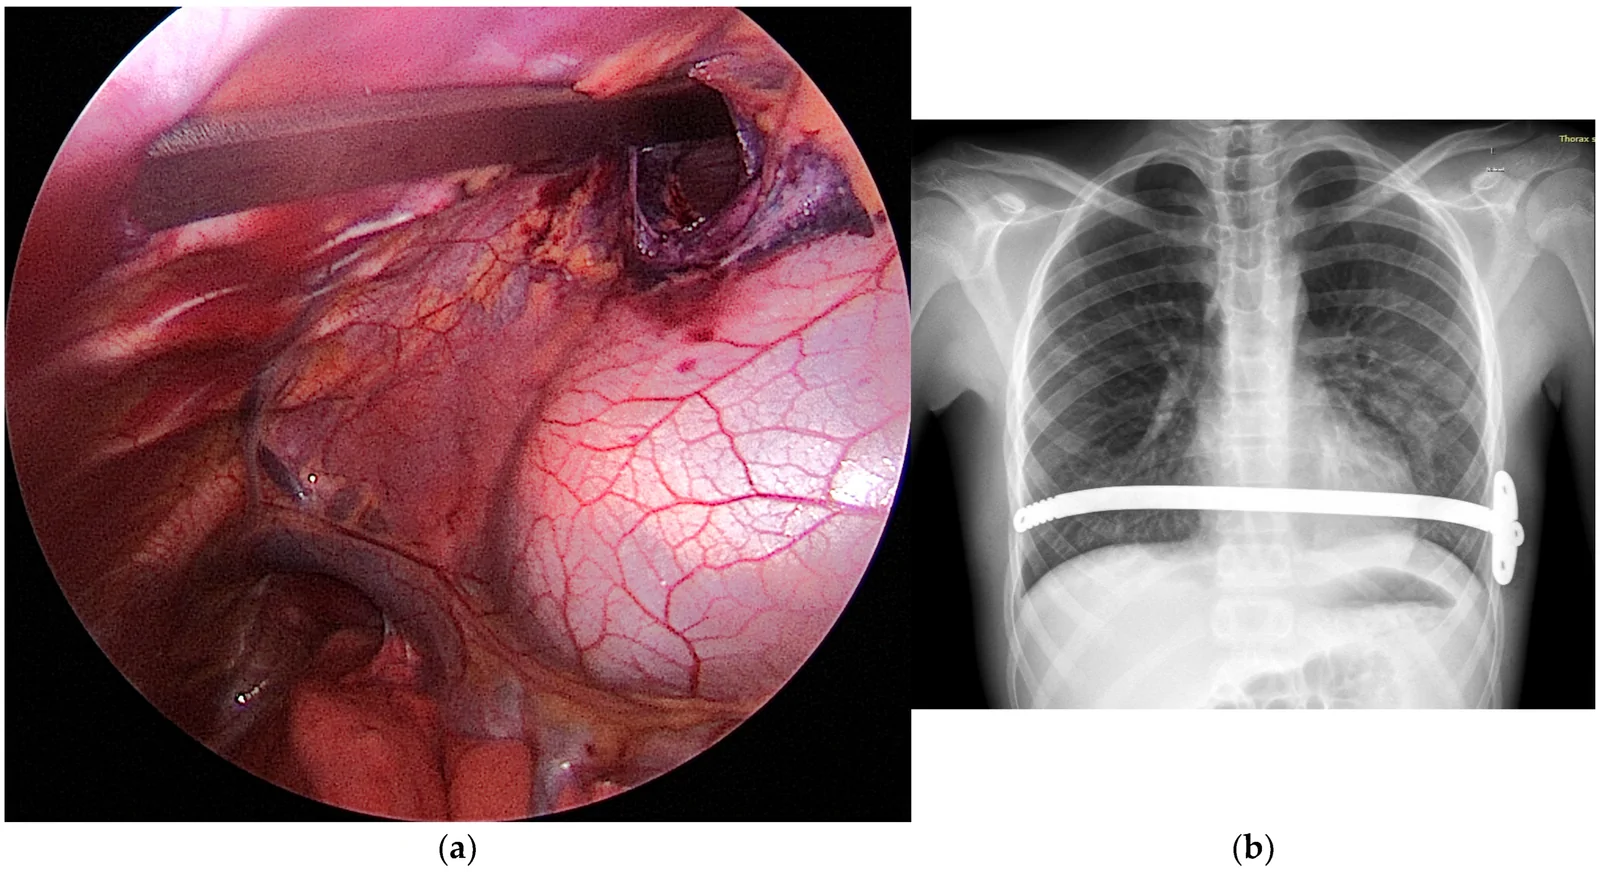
(a) Thoracoscopic view during MIRPE. (b) Chest x-ray after MIRPE.
